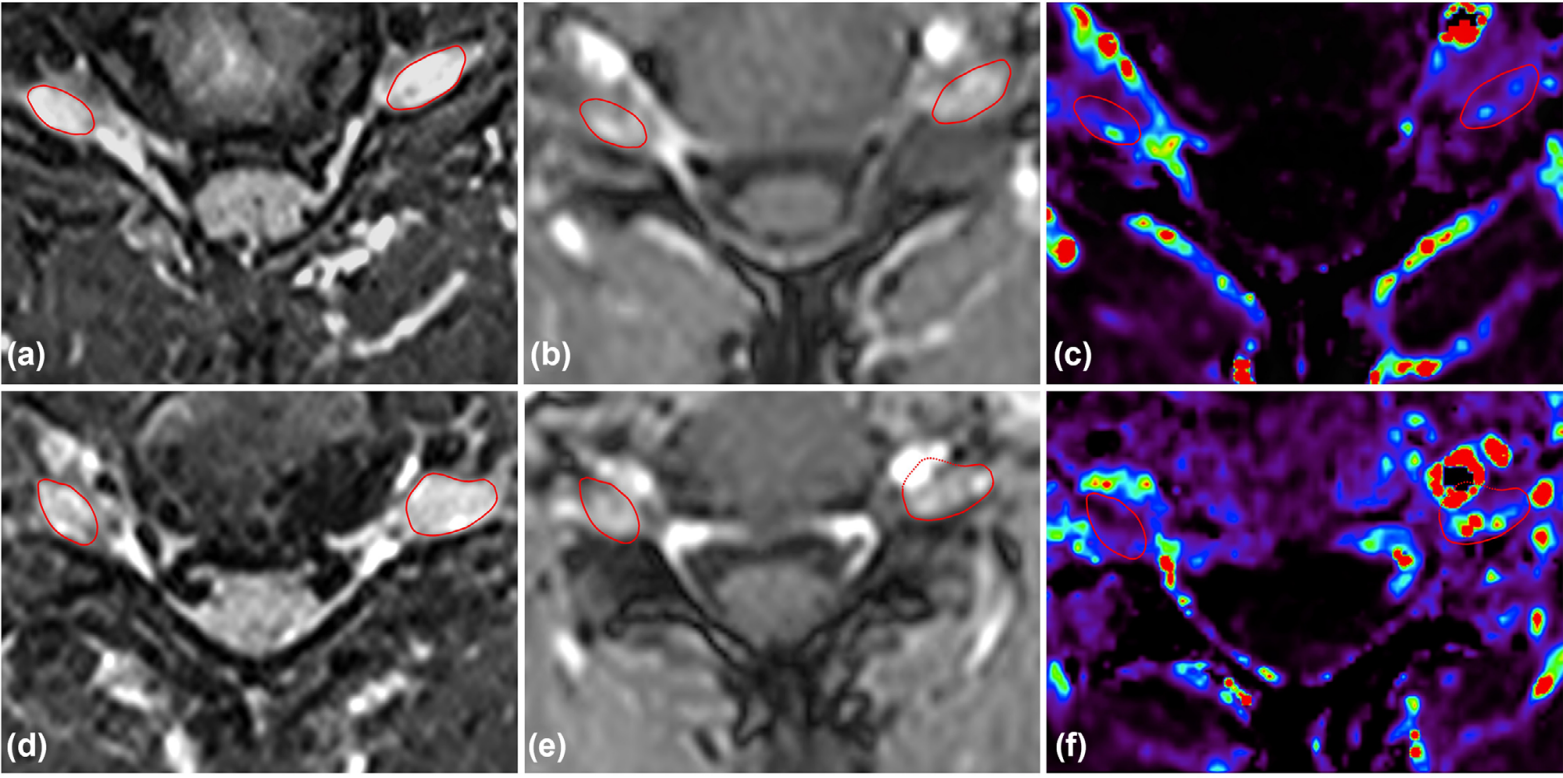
Perfusion magnetic resonance imaging (MRI) images of C7 dorsal root ganglia (DRG). (a–c) T2‐weighed images, gadolinium‐enhanced T1‐weighted images, and pseudo‐color dynamic contrast‐enhanced (DCE) MRI images of C7 DRG in preoperative patients. (d–f) T2‐weighed images, gadolinium‐enhanced T1‐weighted images, and pseudo‐color DCE MRI images of C7 DRG in postoperative patients, right side was the paralyzed side after section. The C7 DRG was circled by red lines. Both the volume and the vascular perfusion of the C7 DRG on the affected side are higher than those on the opposite side.
